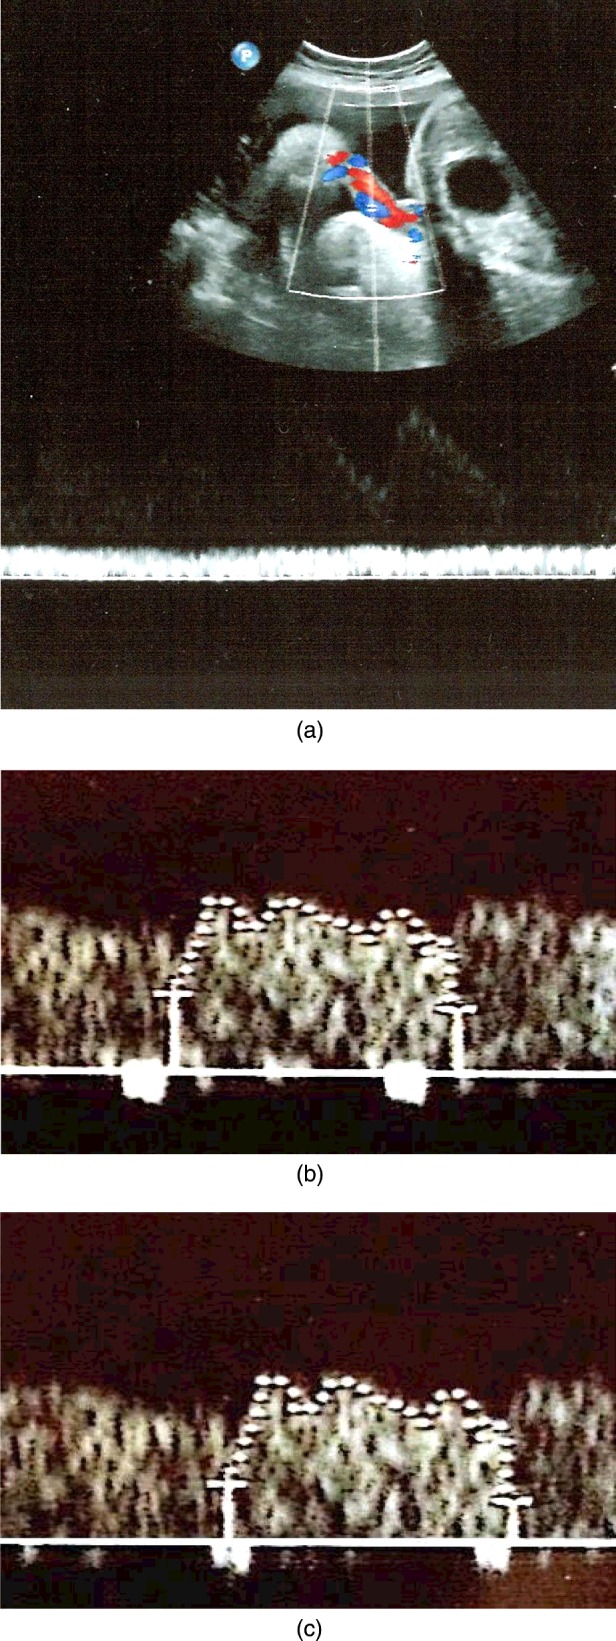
Typical Doppler velocimetric recordings of (a) umbilical vein, (b) inlet of DV and (c) outlet of DV
